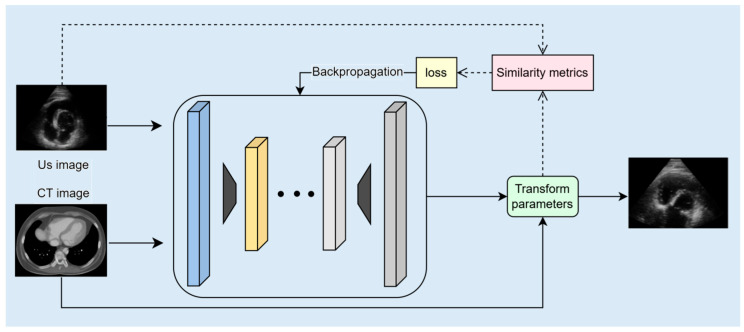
Cardiac CT–ultrasound registration model framework based on unsupervised learning for preoperative surgical planning.
